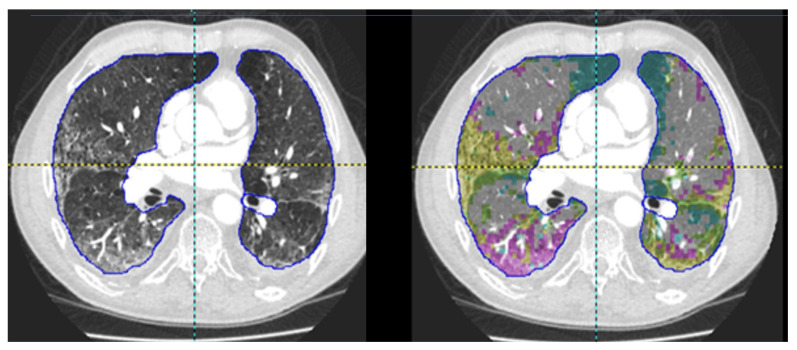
Demonstration of a quantitative CT (QCT) approach (adaptive multiple features method), acquired using PASS software. Different lung parenchymal disease patterns are identified and highlighted. Blue, emphysema/low attenuation pattern. Yellow, fibrotic changes. Pink, ground glass change.
